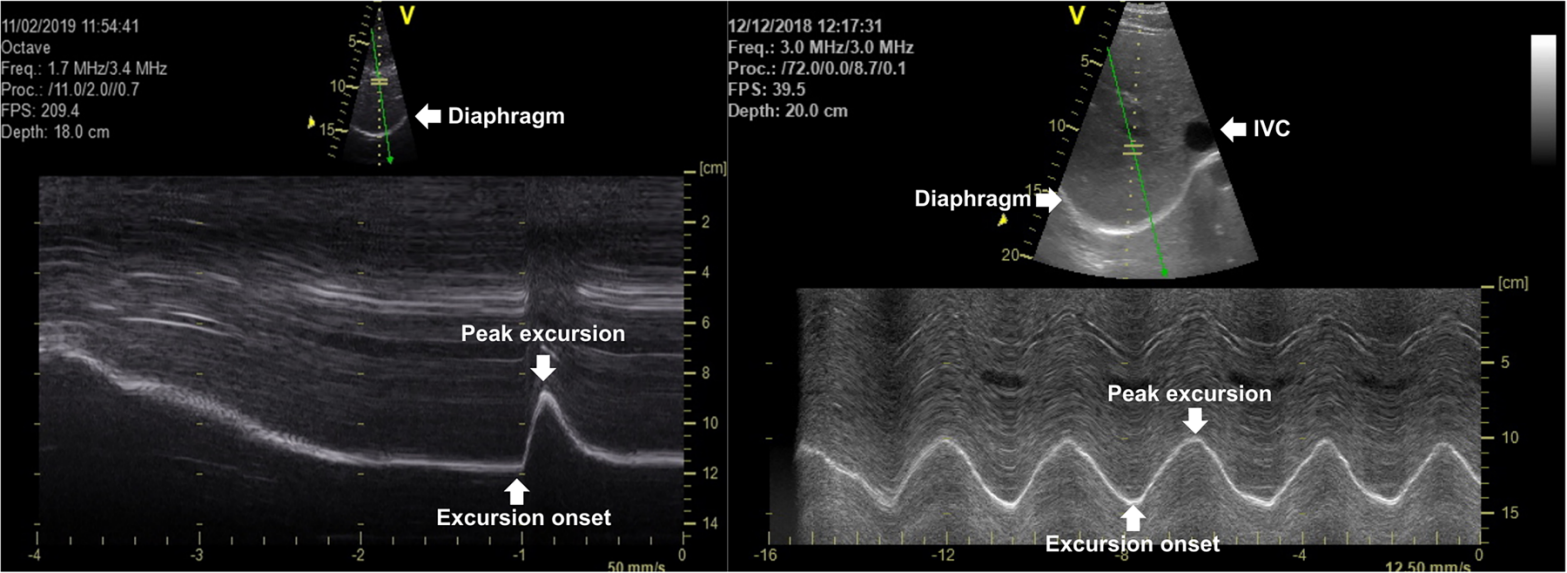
Representative B‐mode (top) and anatomical M‐mode (bottom) ultrasound traces of the right crural hemidiaphragm at baseline (PRE) in response to single ‘twitch’ stimulation of the phrenic nerves at relaxation volume (left panel) and during the final 15 s of CO_2_ rebreathing (end‐tidal partial pressure of CO_2_, 55 mmHg; inspiratory minute ventilation, 32 L/min) (right panel). B‐Mode was used initially to obtain the best diaphragmatic delineation, with the inferior vena cava (IVC) as a landmark to ensure consistency in the positioning of the ultrasound transducer. In anatomical M‐mode, diaphragm excursion (*y*‐axis) and excursion time (*x*‐axis) were measured for the determination of excursion velocity, work and power.
